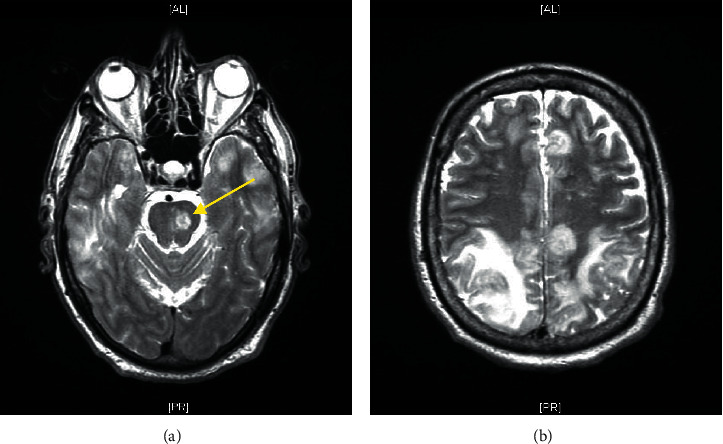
MRI brain with and without contrast showing (a) new left brain stem lacunar infarct (yellow arrow) and (b) worsening sulcal and cisternal T2 hyperintensity and nodular pial enhancement suggesting progression of meningitis. New rather extensive symmetric vasogenic edema due to progressive cerebritis.
